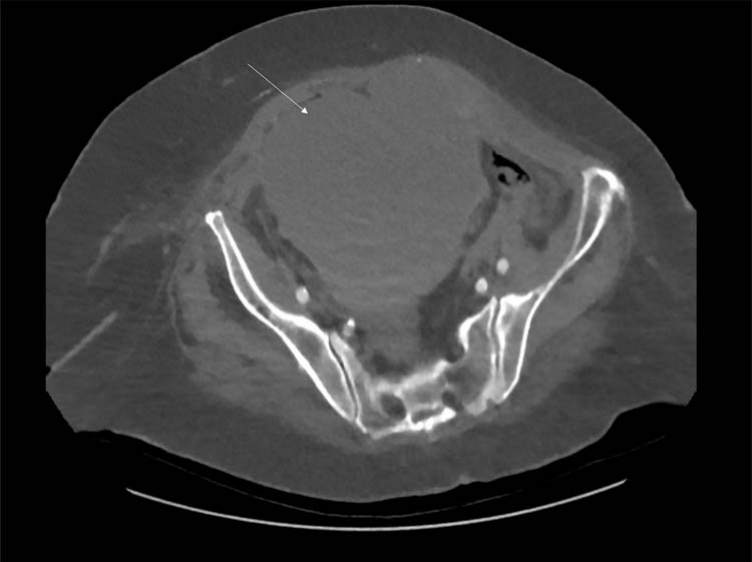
Large rectus sheath haematoma on computed tomography (white arrow).
